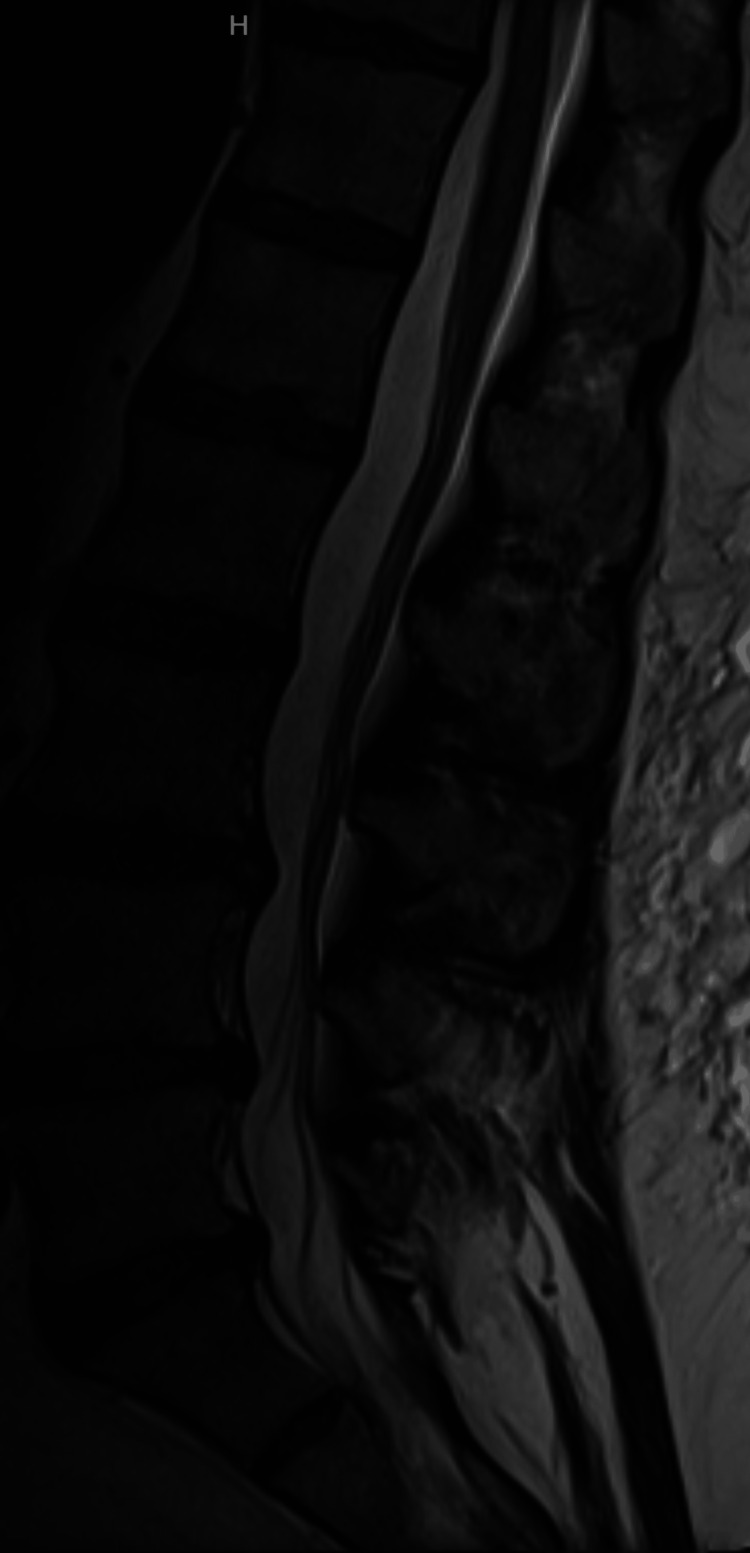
MRI of the lumbar spine is unremarkable
